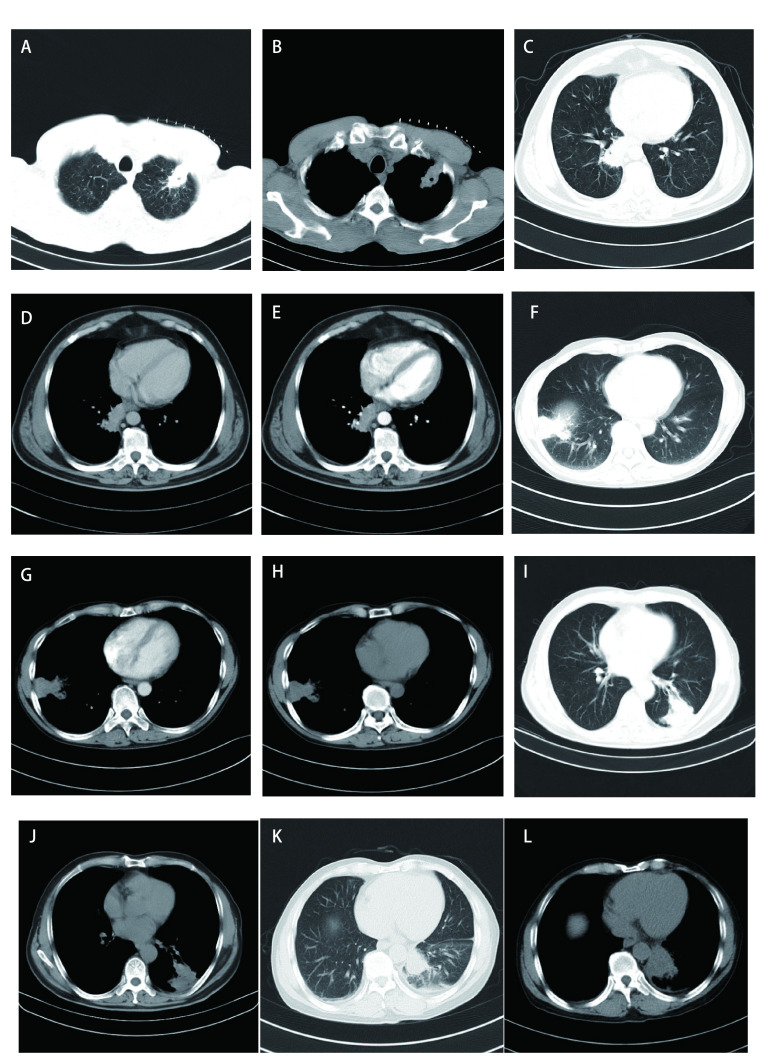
胸部CT：病灶边界不清楚。A、B为同一患者：左肺上叶见不规则片状密度增高影，内有小片低密度区；C、D、E为同一患者：右肺下叶后基地段脊柱旁见团块状密度增高影，呈分叶状，周围见短毛刺，增强扫描明显强化；F、G、H为同一患者：右肺下叶见一不规则片状高密度影，增强扫描呈不均匀轻中度强化；I、J为同一患者：左肺下叶背段及后基底段见一三角形软组织肿块，密度欠均匀，边界不清，内有多发点状、片状低密度影；K、L为同一患者：左肺下叶背段见一软组织肿块，最大截面约54 mm×49 mm，边界不清，密度欠均匀。 Chest CT: lesion boundary is unclear. A and B are the same patient: An irregular high density flake shadow with small low density area is showed in the left upper lobe; C, D and E are the same patient: CT showed a high density mass shadow in the posterior base segment of the right lower lobe, with unclear boundary, lobular shape, short burr around, enhanced CT scans significantly enhance; F, G and H are the same patient: An irregular high density flake shadow is showed in the right lower lobe, the enhanced scan is unevenly lightly moderately reinforced; I and J are the same patient: A triangular soft tissue lump shadow with multiple dots and flaky low density shadow was found in the dorsal and posterior basal segments of the left lower lobe, the density of lump shadow is uneven, the boundary is unclear; K and L are the same patient: A soft tissue lump shadow in the dorsal segment of left lower lobe, the maximum cross section size is about 54 mm×49 mm, the boundary is unclear and density is uneven.
